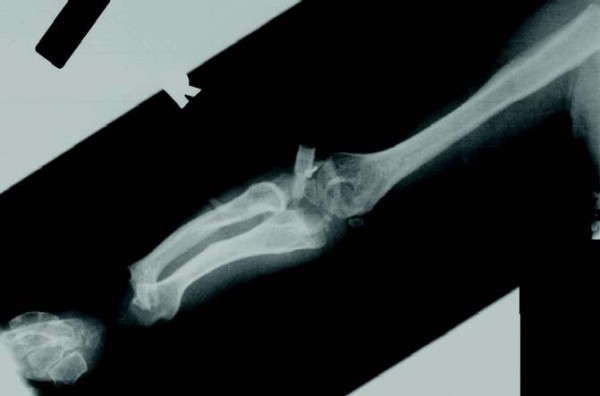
Rapidly progressing 9-year old female MPS VI patient: radiograph of arm showing ulna radius and humerus.
